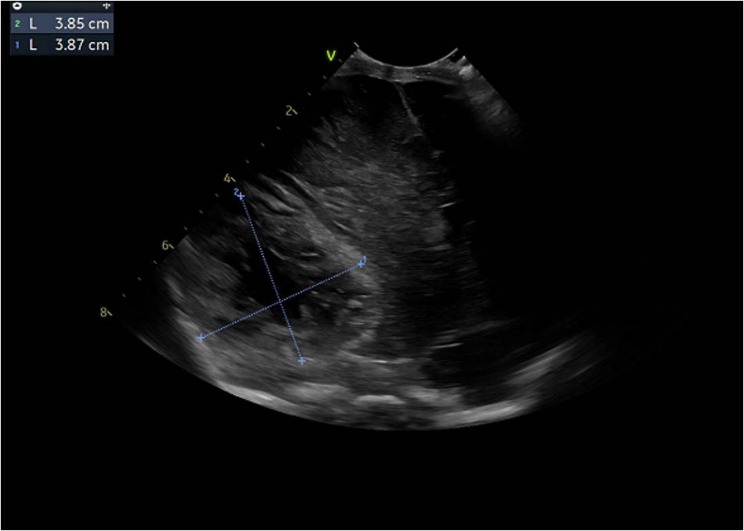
Cranial ultrasound (coronal view) demonstrating suspicious 3.85 × 3.87 cm area indicative of haemorrhage
